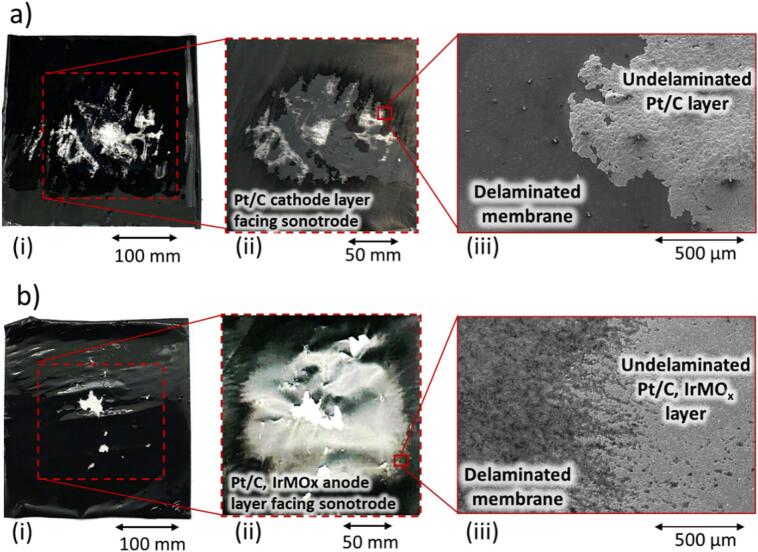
Delamination of fuel cell CCM induced by ultrasonic cylinder sonotrode. Images of (a) the Pt/C cathode side and (b) the Pt/C, IrMO_x_ anode side of a CCM sample after ultrasonic delamination in water (10 % power, 1-second sonication time, 5 mm sonotrode-sample distance). (i) Initial sample, (ii) sample after drying (image adjusted for brightness and contrast), and (iii) corresponding SEM image of the delamination interface. For the detailed elemental analysis of regions indicated in (iii), refer to Fig. S7.
